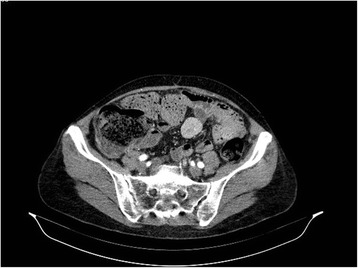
A CT showing tumour of the mesentery with prominent contrast enhancement.
